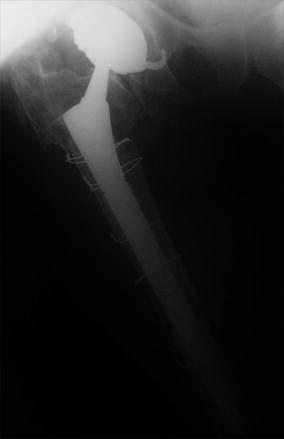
Radiograph after the first operation reveals good relationship of the total hip prosthesis with acetabular and femoral bones
